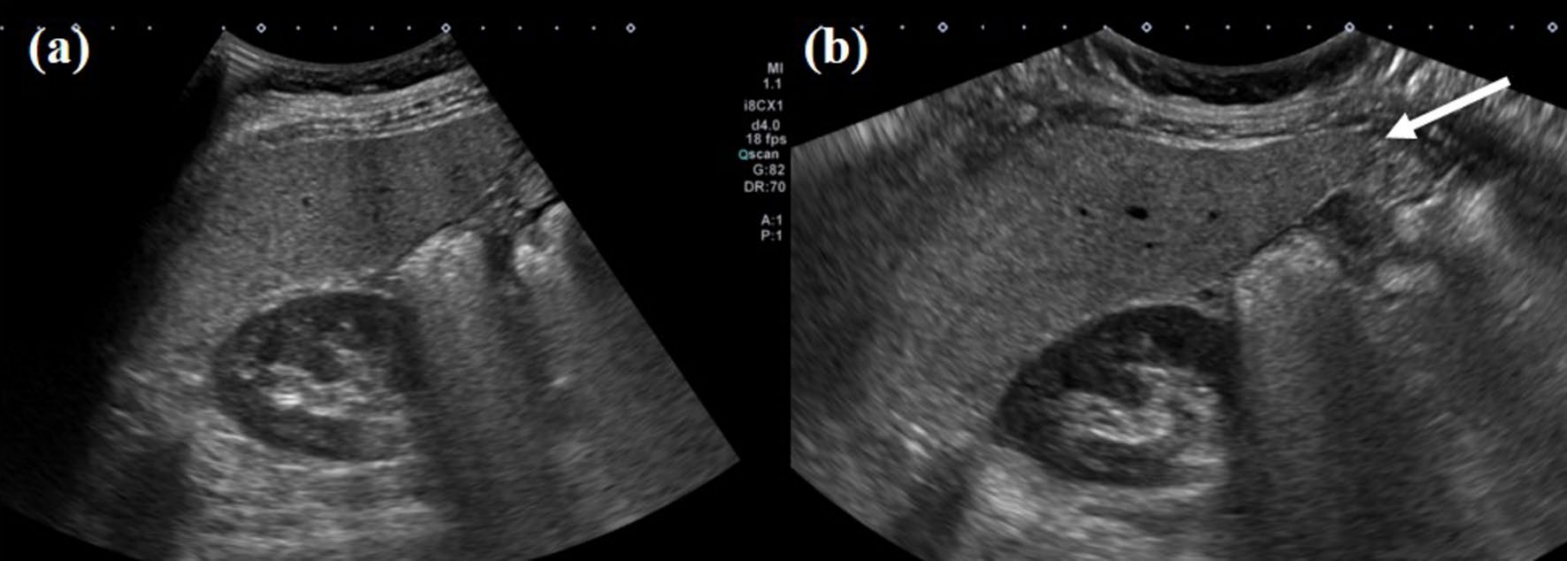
Ultrasound (US) images acquired from a 62-year-old female patient with fatty liver. a Intercostal scan of the liver right lobe using a routine view mode, demonstrating limited coverage that does not include the segment VI tip of the liver. b Intercostal scan of the liver right lobe using a wide view scan mode, providing complete coverage of the segment VI tip of the liver (arrow)
